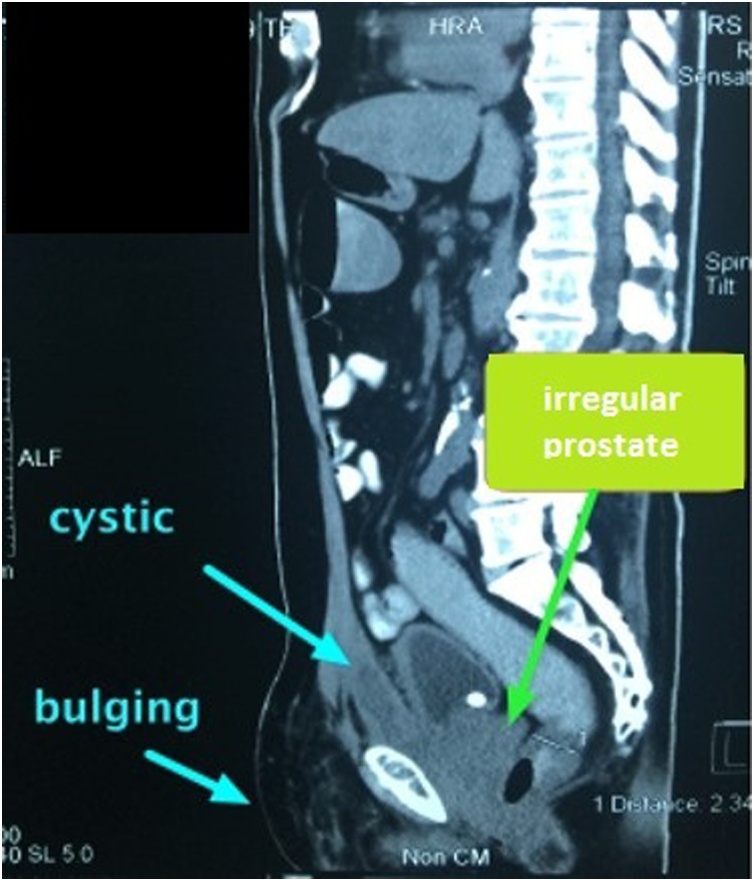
3-month follow-up CT Scan axial view showed mass on the prostate.
